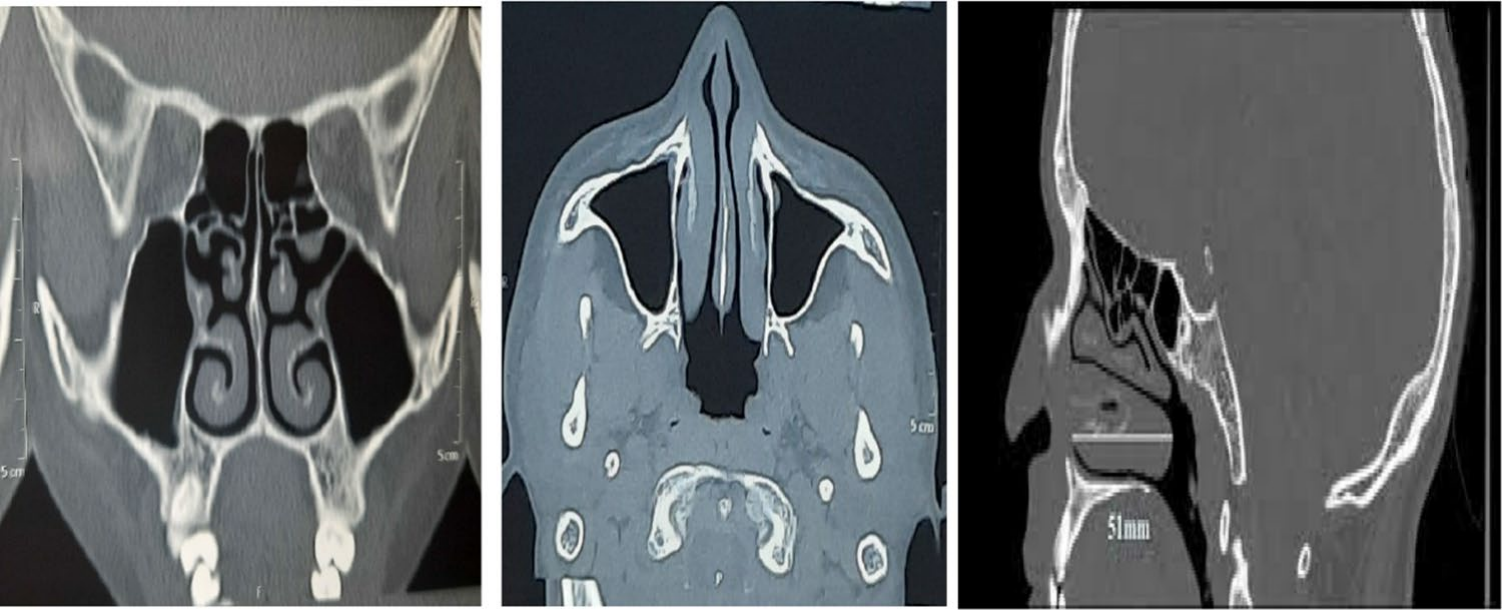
CT PNS (coronal, axial and sagittal sections) showing Bilateral Inferior turbinate hypertrophy (ITH)
